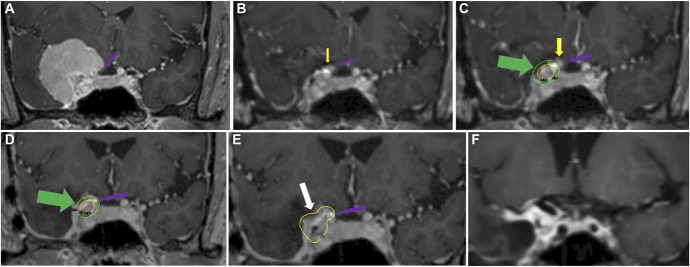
A, Preoperative Gd-enhanced T1W coronal MRI image showing a clinoidal meningioma with cavernous sinus invasion and compression of the optic nerve (purple arrow). B, Immediate postoperative gadolinium-enhanced T1W MRI showing the fat graft (yellow arrow) between the optic nerve (purple arrow) and the residual tumor. C, Hypothetical single-fraction dosimetry with SRS (on immediate postoperative MRI): The green dosimetric curve shows the limit of the 8 Gy dose that passes through the fat graft and stays at a distance from the optic nerve. D, MRI at 7 months after surgery (with further fat resorption): The green dosimetric curve of 8 Gy is in contact with the optic nerve (due to fat shrinkage), which did not allow single-fraction treatment. E, MRI at 7 months after the surgery showing the yellow dosimetric curve of the tumor and the tumor (white arrow) treated by hfRS. F, MRI at 2-year follow-up after hfRS with further shrinkage of the graft and tumor. hfRS, hypofractionated radiosurgery.
